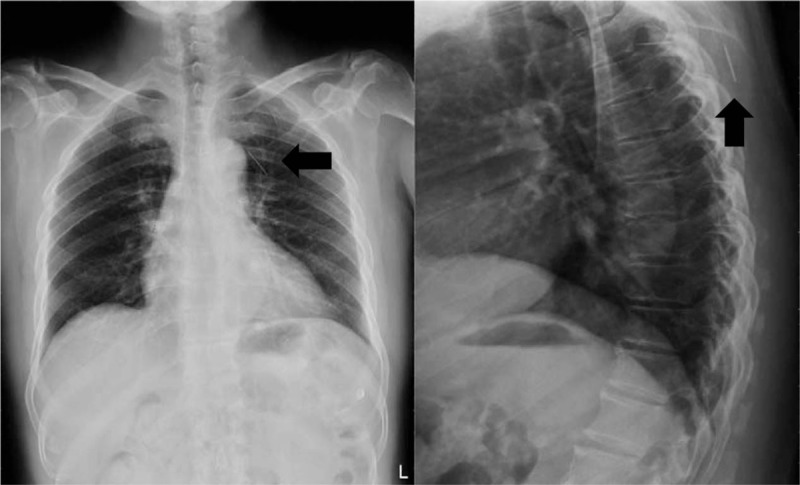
Chest X-ray scan showing foreign bodies (fragments of needle) in the spinal canal of T4-5 and upper back.
